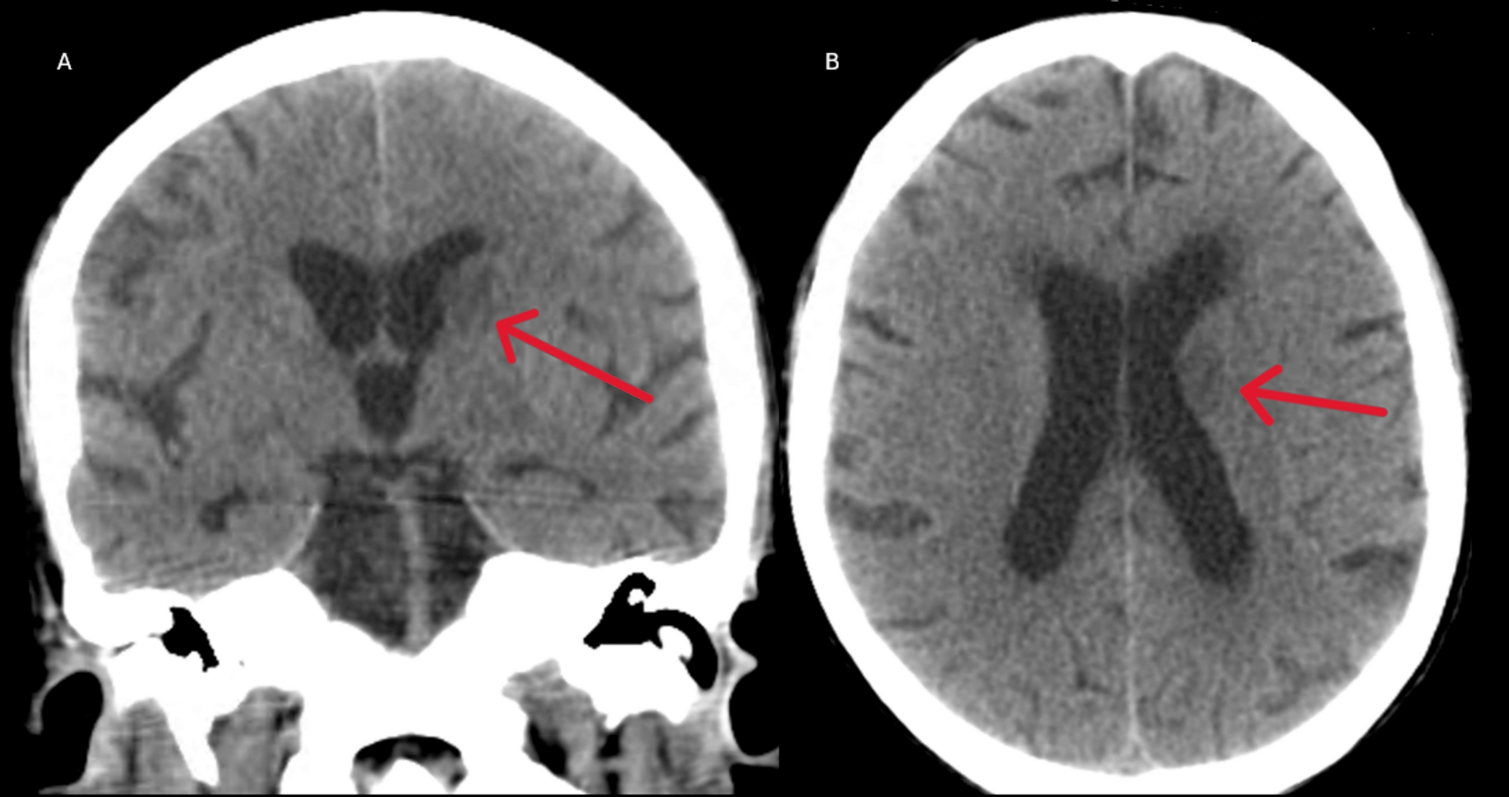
Coronal (A) and axial (B) view of the cerebral CT scan showing the hypodense area on the left gangliocapsular region (red arrows) CT: computed tomography
